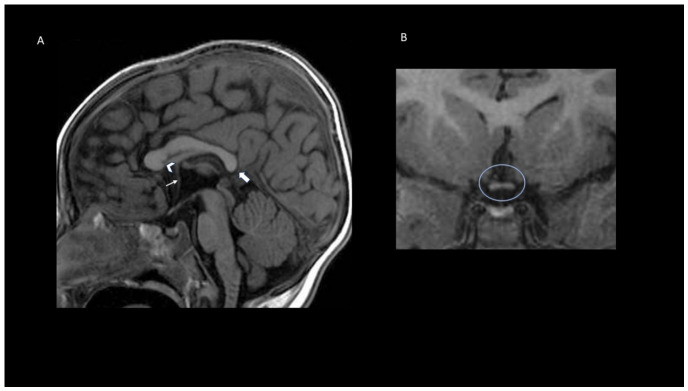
(A) Sagittal T1-weighted image demonstrates a reduced anteroposterior diameter of the corpus callosum, hypoplasia of the splenium (thick arrow), poor visualization of the rostrum (arrow-head), and marked thinning of the anterior commissure (thin arrow). (B) The circle in coronal T1-weighted image demonstrates mild thinning of the optic chiasm.
